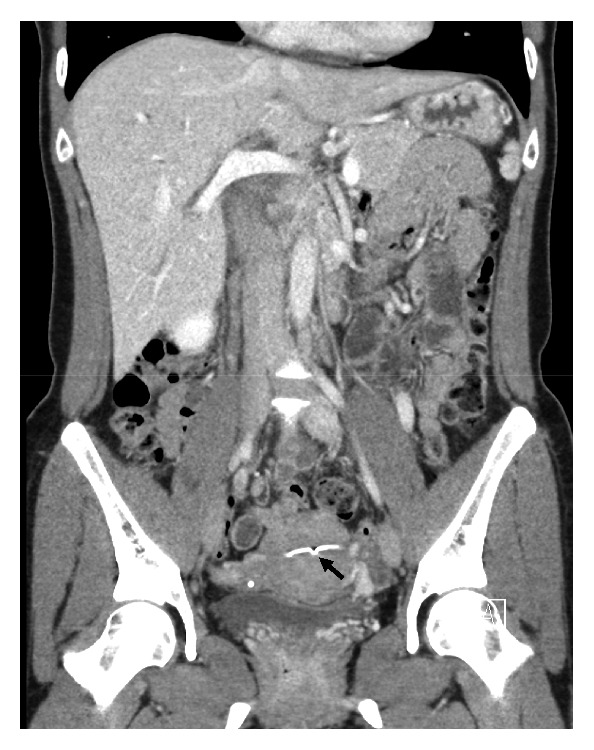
Case # 2, Mirena hormonal intrauterine device in uterus (arrow), CT of Abdomen.
